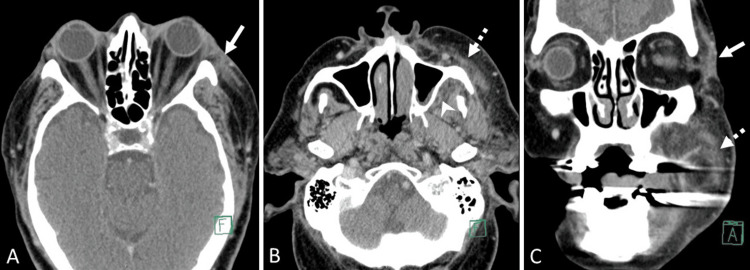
Contrast-enhanced CT of the paranasal sinuses (A, B) Axial and (C) coronal images show mild soft tissue thickening and fat stranding in the left buccal space (dashed white arrow), retroantral regions (solid white arrowhead), and left inferior periorbital soft tissues (solid white arrow). These findings are suggestive of cellulitis.
